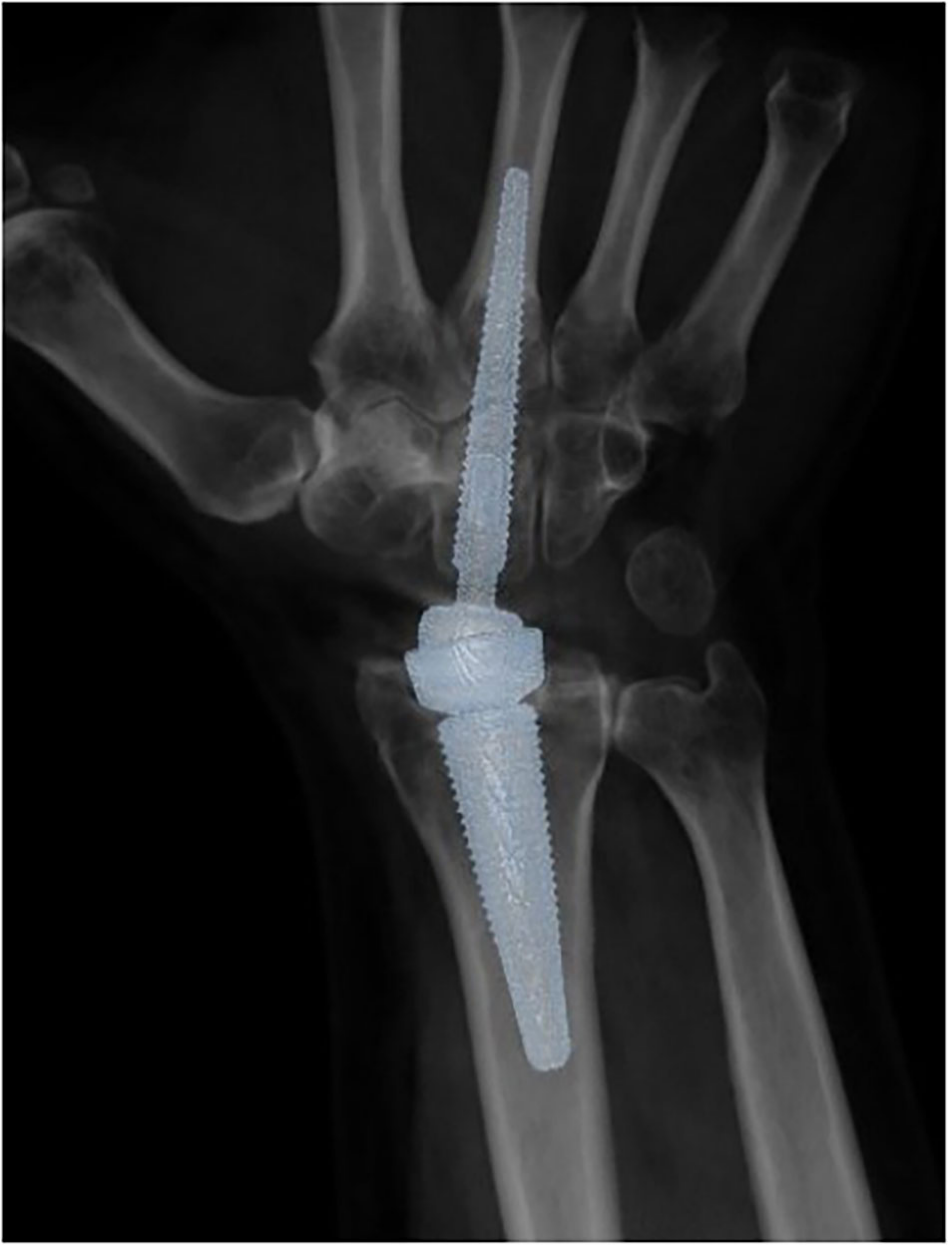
Three-dimensional reconstruction of a Motec® Wrist Joint Prosthesis acquired using photon-counting detector computed tomography (polyenergetic reconstruction)
